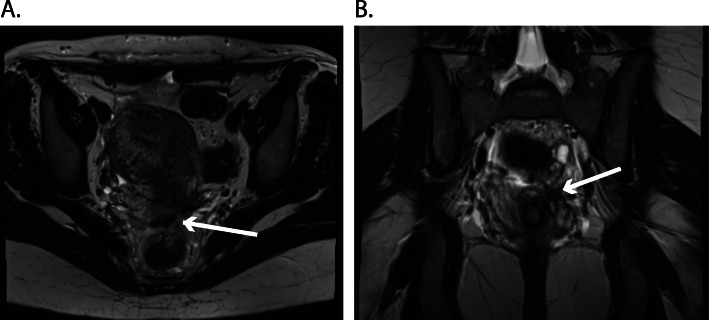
Abdominal magnetic resonance imaging (MRI) two months after the delivery a. T2 weighted transverse MRI and b. T2 weighted coronal MRI showed an endometriosis node 3x2cm (white arrow) adjacent to the septum rectovaginale with expansion to the left ovary and in close proximity to the sigmoid without infiltration according to an Enzian score A2, B1, C1
